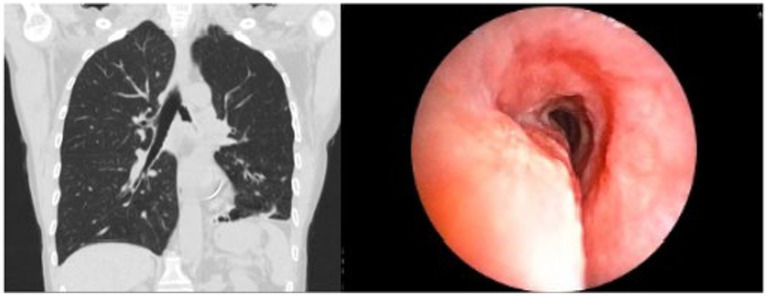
Case 1: Coronal CT scan (left) showing severe stenosis of the left main bronchus, and corresponding bronchoscopic view (right) demonstrating the endoluminal narrowing at the same anatomical site.
